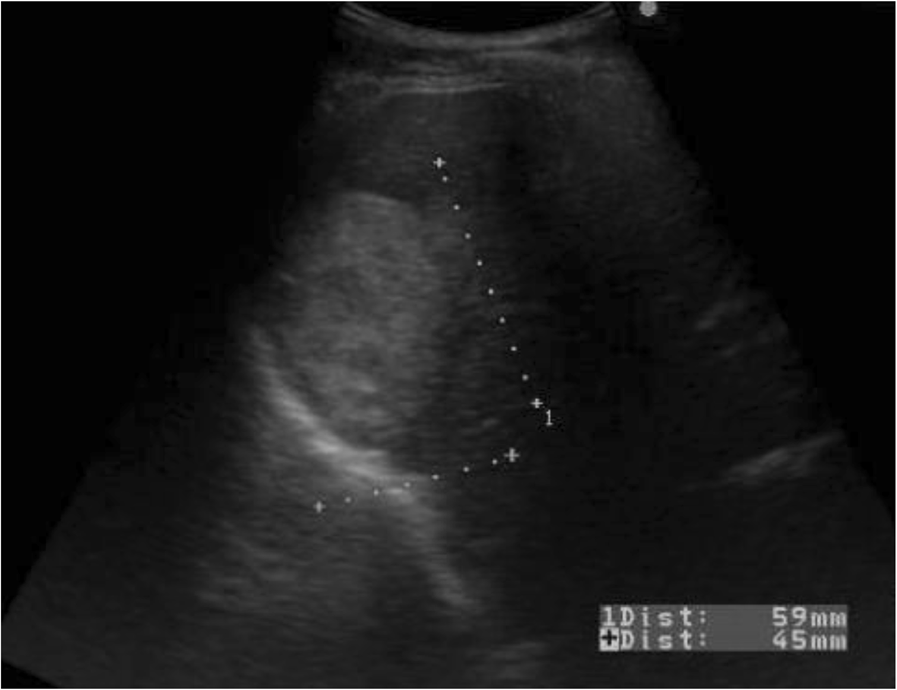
Abdominal ultrasonography (US). US showed a heterogeneously hyperechoic mass in segment 7 of the liver.
